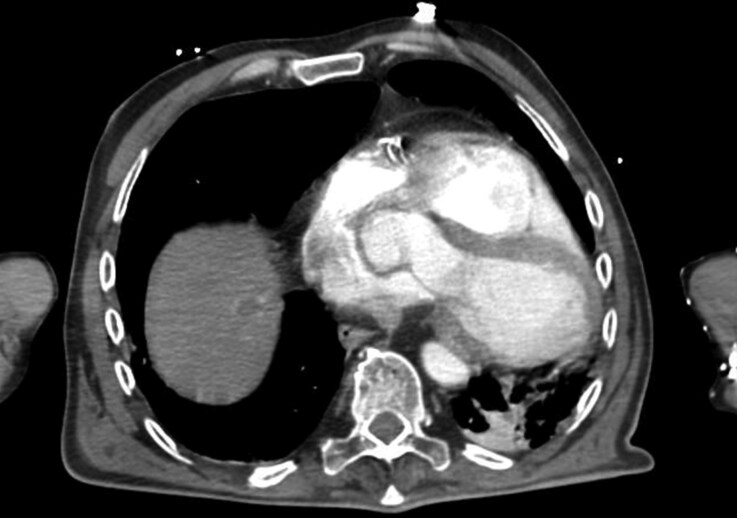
Cardiac CT scan of heart levorotation.
